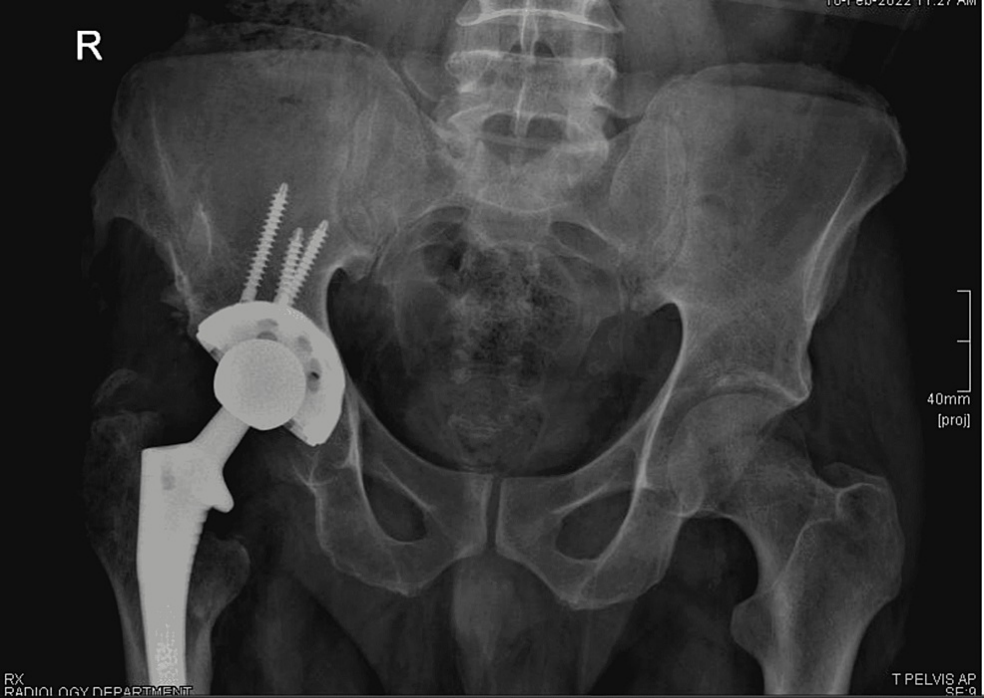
Pelvic x-ray after total hip replacement.
